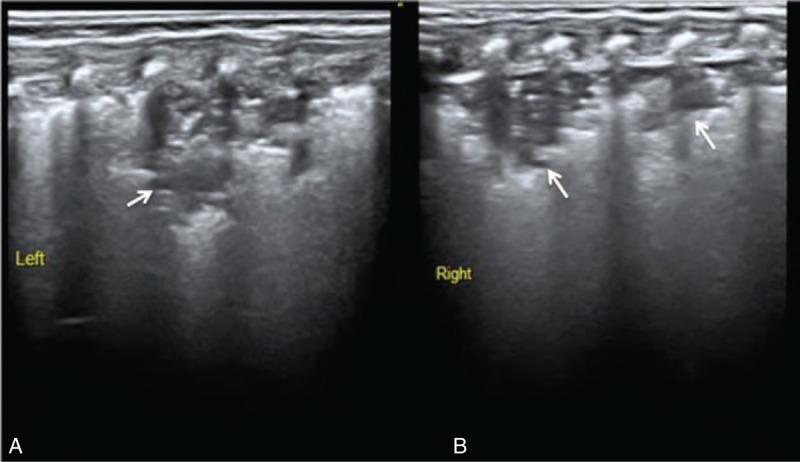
Pneumonia. GA 29^+2^ weeks, birth weight 1200 g, Apgar score 8-10-10 points/1-5-10 min. This patient was maintained on a ventilator because of RDS and was diagnosed with BPD clinically because oxygen therapy was required after 58 days of life. A lung ultrasound showed large consolidations with irregular edges, as well as air bronchograms bilaterally (A—left lung, B—right lung) (arrows). The pleural line was either blurred or disappeared, and the A-line disappeared. This infant was diagnosed with bilateral pneumonia, which was the reason for oxygen dependence. BPD = bronchopulmonary dysplasia, GA = gestational age, RDS = respiratory distress syndrome.
